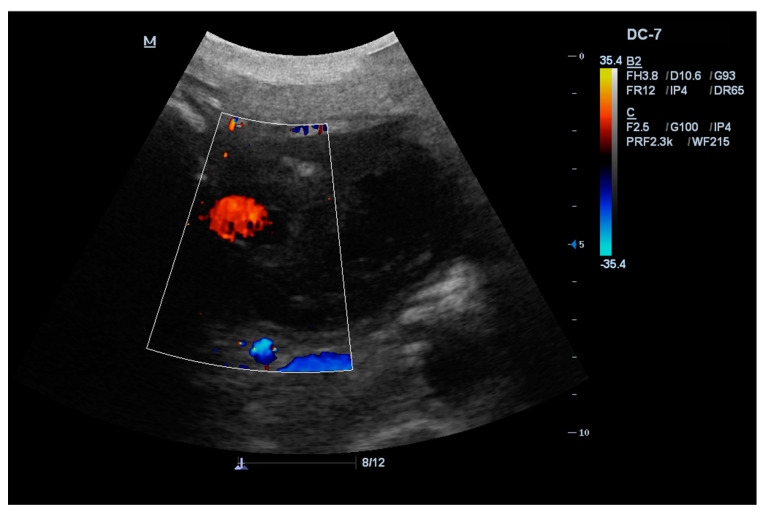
Abdominal ultrasound with color Doppler shows a large hypoechoic cystic lesion in the pancreatic head with internal turbulent blood flow.
